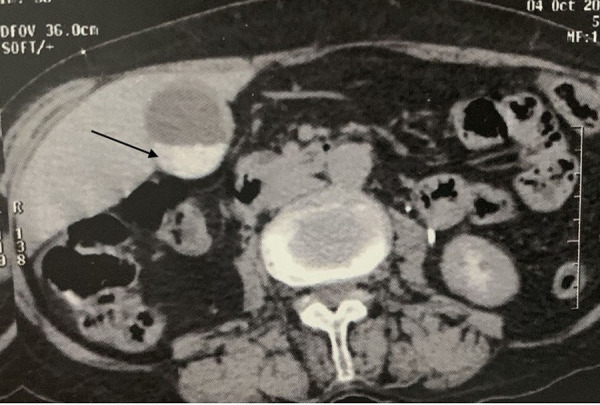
abdominal computed tomography scan showing a gallstone
